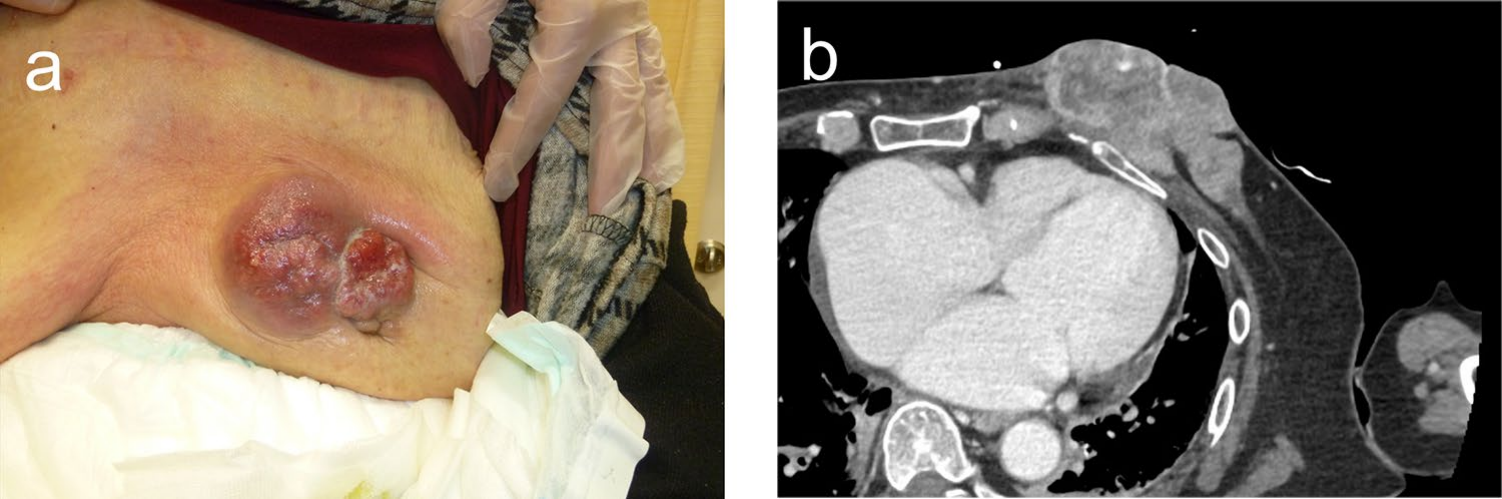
Initial presentation of the breast mass. a Physical examination revealed a 60 × 50 mm nodular mass occupying the left breast, accompanied by surrounding erythema. b A computed tomography (CT) scan showed a centrally necrotic, peripherally contrast-enhanced soft tissue mass centered in the left breast
